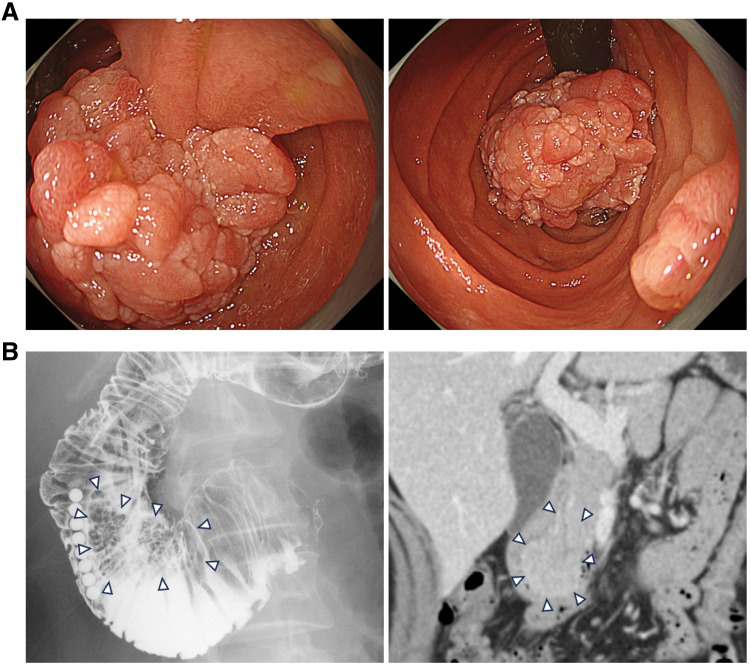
Preoperative endoscopic and radiological findings. (A) Esophagogastroduodenoscopy showing a 40-mm villonodular tumor in the 2nd portion of the duodenum (left: close-up view; right: tumor occupying the lumen). (B) Upper gastrointestinal contrast study demonstrating a filling defect (left, arrowheads) and CT revealing a corresponding lesion without local invasion or distant metastasis (right, arrowheads).
